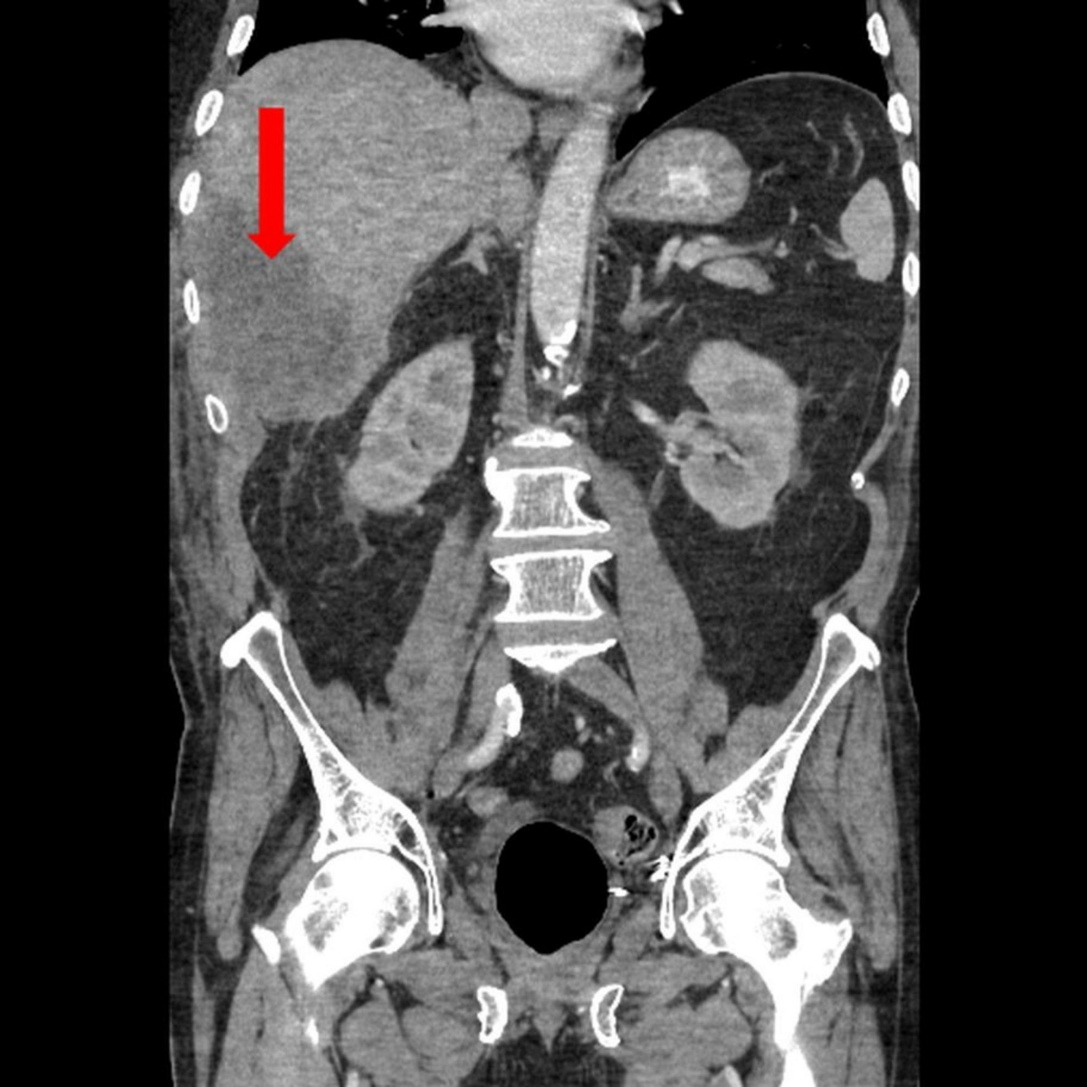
Whole body computed tomography scan showing a large 19-cm sub-diaphragmatic and a right retro-peritoneal abscess inferior and posterior to the right hepatic lobe (red arrow)
